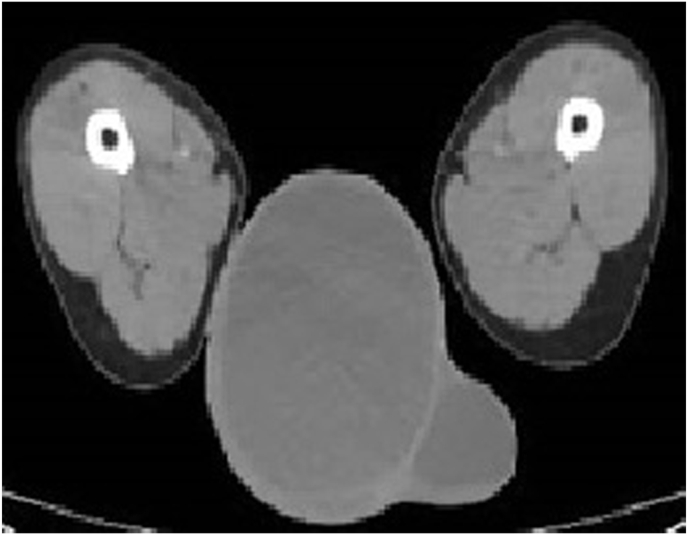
CT scan showing testicular swelling.
